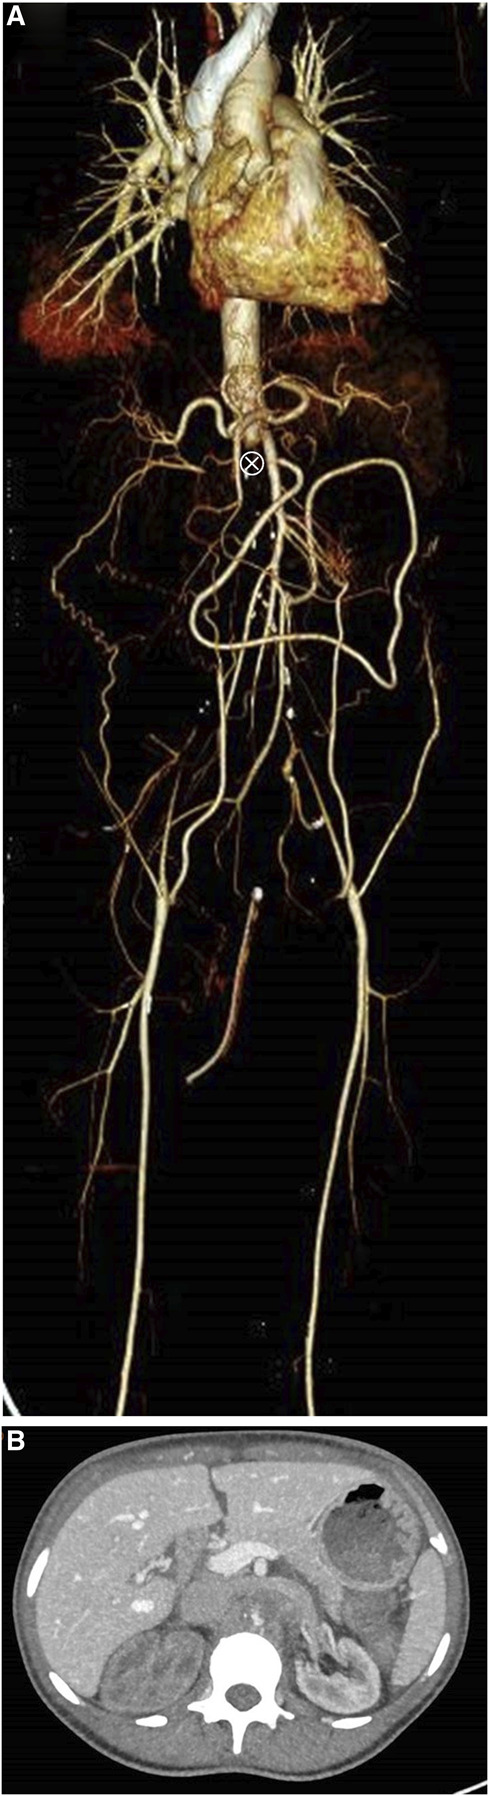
Contrast-enhanced CT scan (3D reconstruction) showing acute aortic occlusion juxta-superior mesenteric artery. (A) Aortic occlusion juxta-superior to the mesenteric artery (marked with an ⊗) along with collateral vessels in the abdominal wall on contrast-enhanced CT scan with 3D reconstruction. (B) A left kidney with contrast enhancement and right kidney without enhancement suggesting lack of kidney perfusion. CT, computed tomography.
